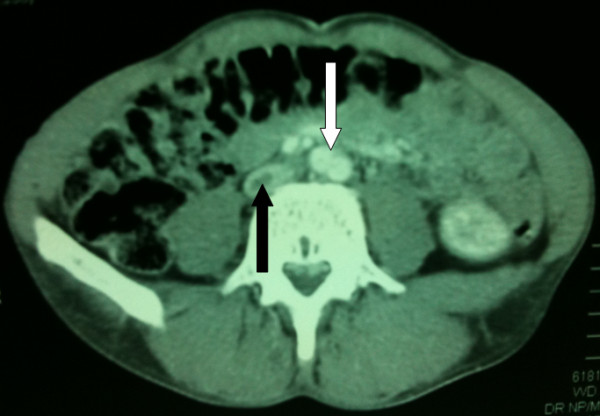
Contrast computed tomography of the pelvis demonstrating thrombosis (black arrow) within the lower inferior vena cava, just before the abdominal aortic bifurcation (white arrow).
